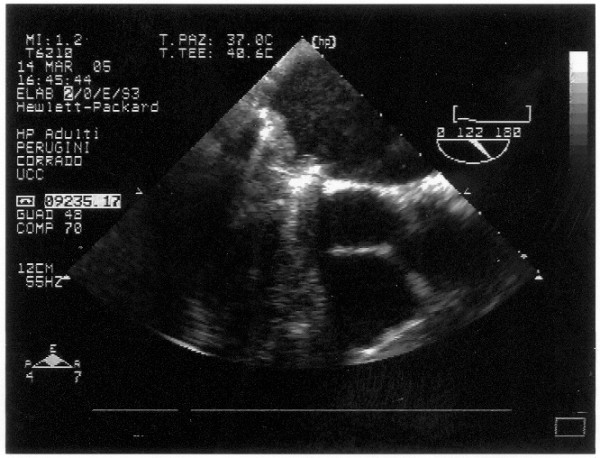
Transesophageal echocardiography: 2D evidence of mitral prosthesis valve thrombosis.
